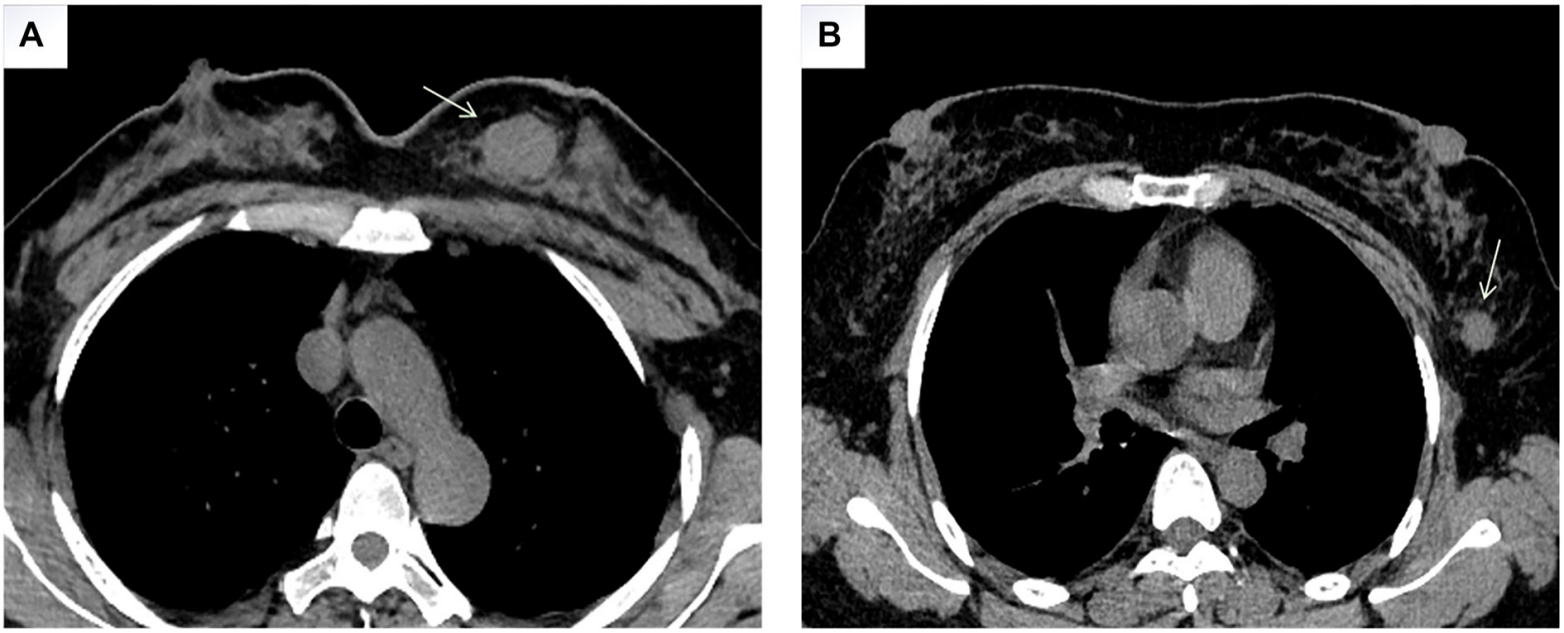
An example of chest CT images that completely include breast tissue. Arrows point to the locations of breast masses. (A) Represents a benign breast mass (breast fibroadenoma); (B) Denotes a malignant breast mass (early non-specific invasive breast cancer). Abbreviation: CT: Computed tomography.
